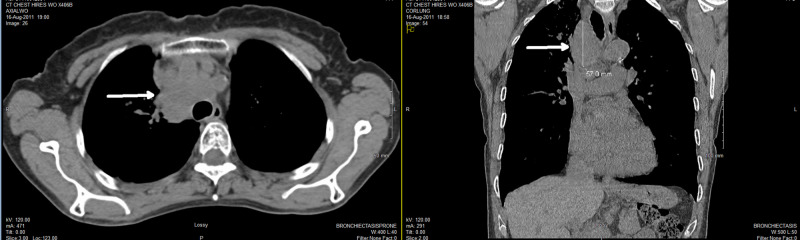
Initial diagnostic CT of the chest showing large masses in the right upper lobe lung and mediastinum
